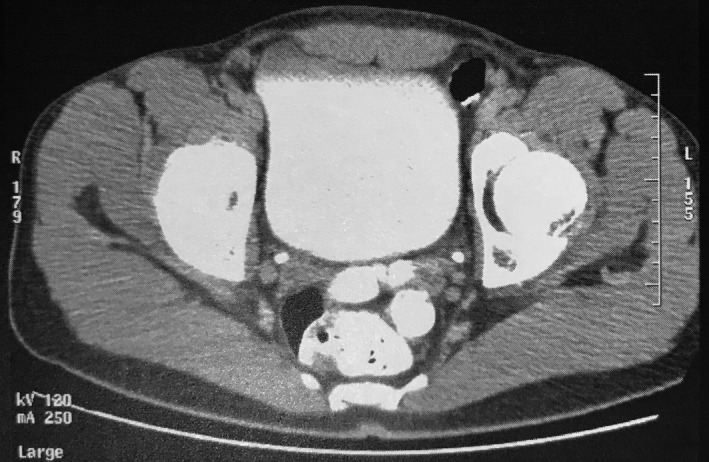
Axial view of second CT Urography (CTU) 6 mo later, indicating the absence of papillary lesion inside the bladder
